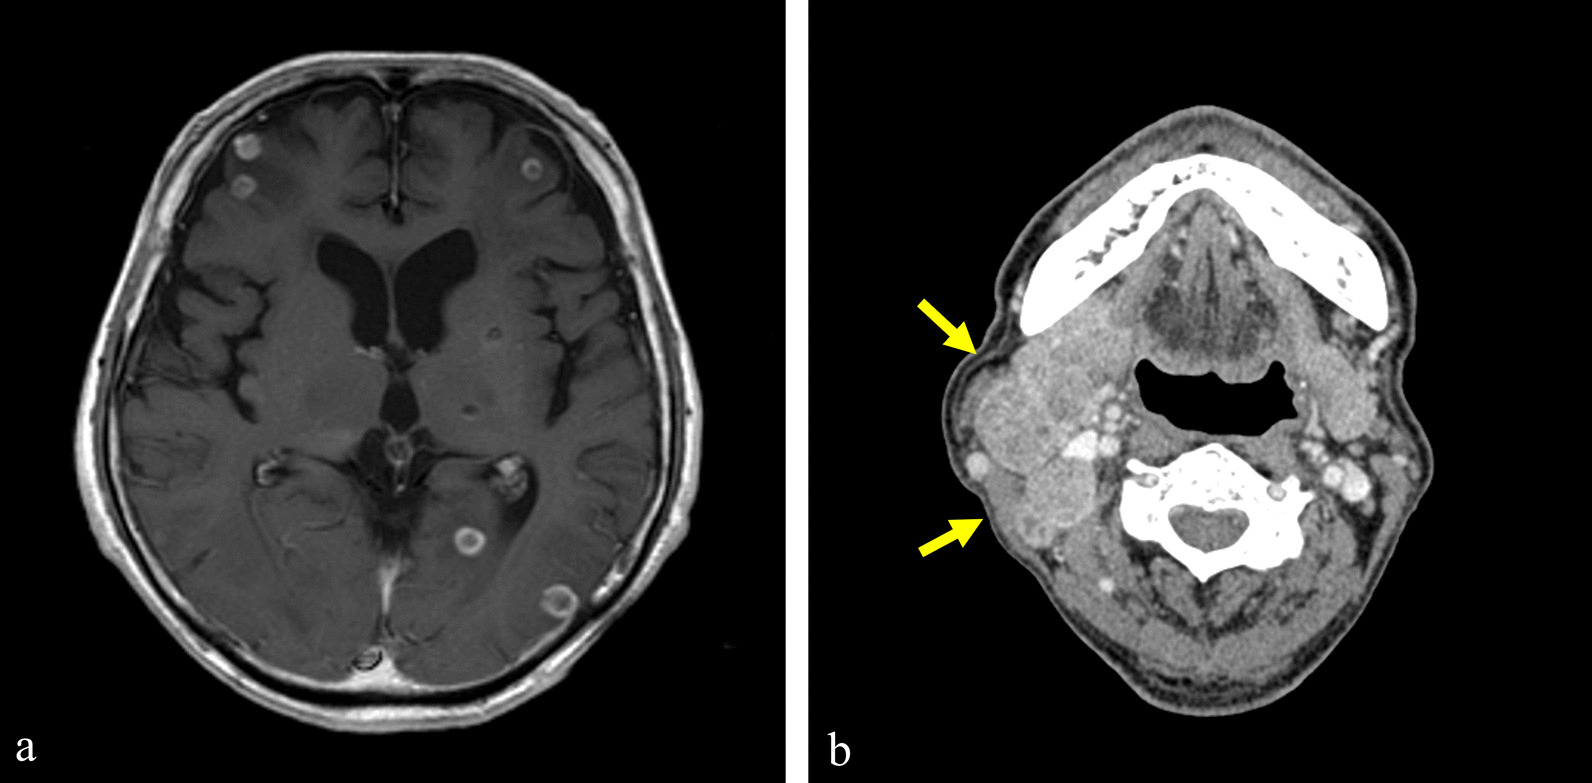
Fifteen months after whole-brain radiotherapy, brain magnetic resonance imaging showed multiple brain metastases (a) and computed tomography showed right cervical lymph node metastases (yellow arrows) (b)
